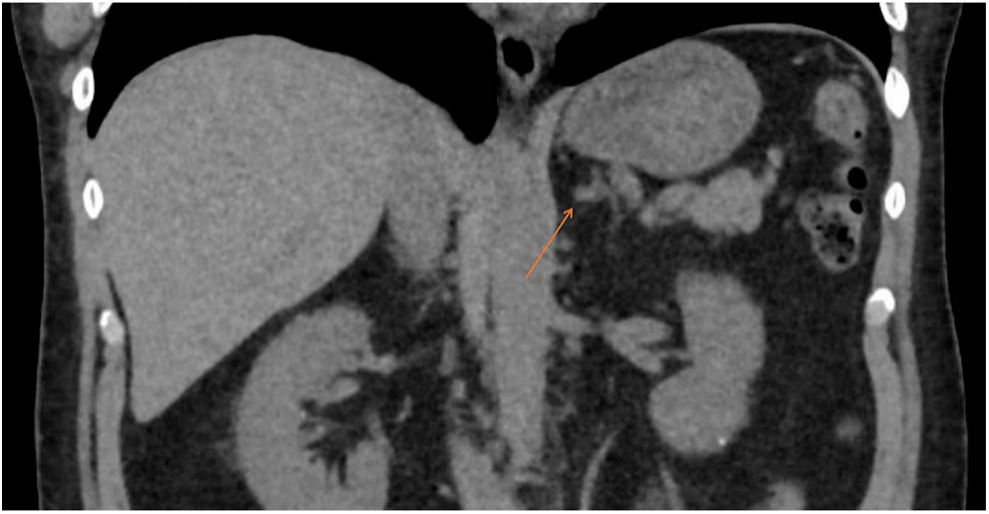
Coronal view of CT of the adrenals showing a 1.2 cm left adrenal nodule of 35 houndsfield units.
